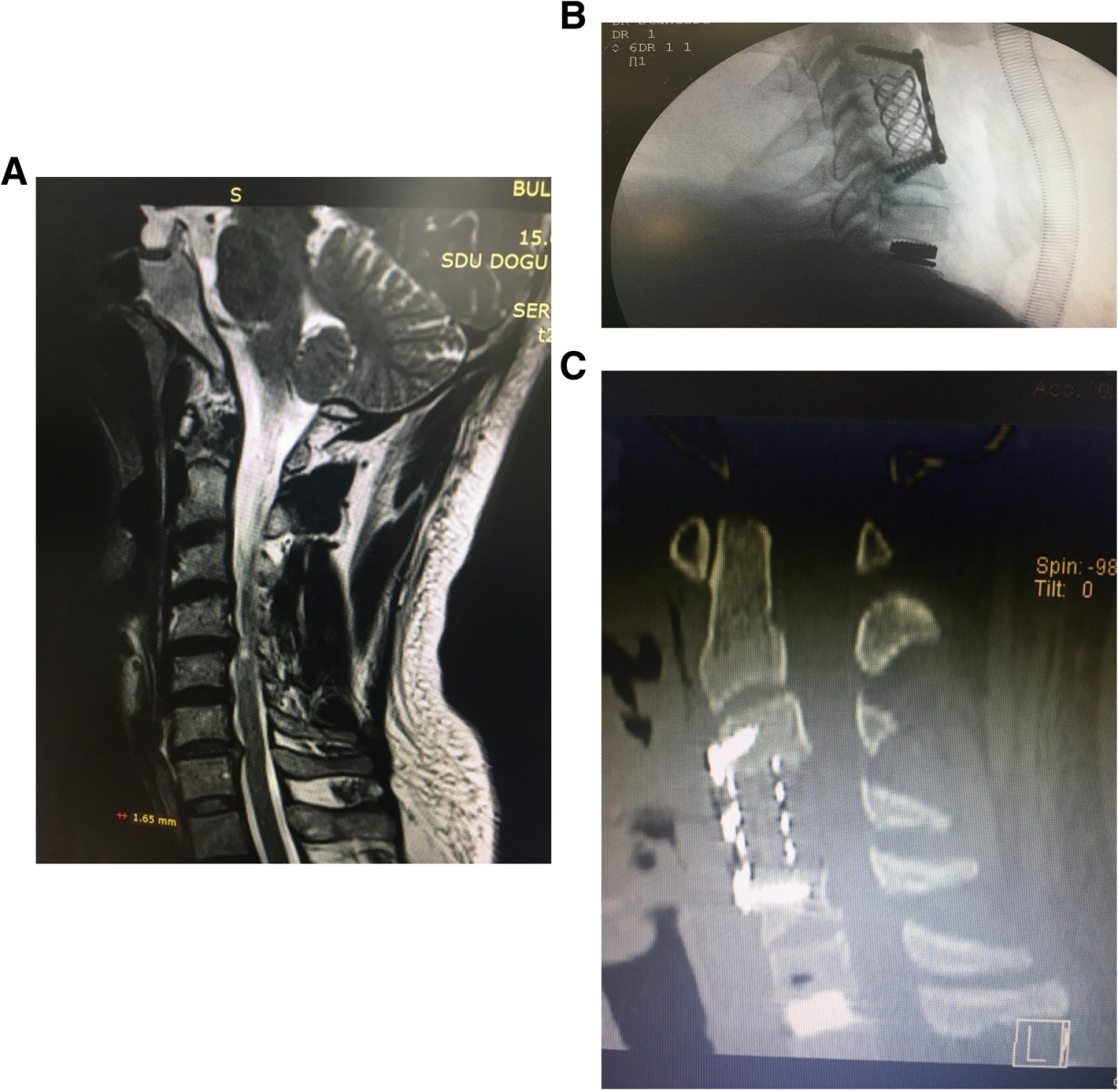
a Magnetic resonance images of spinal stenosis and myelopathy involving C4–C5 levels. b Plain radiograph of corpectomy for C4, discectomy, and disc prosthesis for C6–C7. c Computerized tomography scans of corpectomy for C4 and discectomy and disc prosthesis for C6–C7
